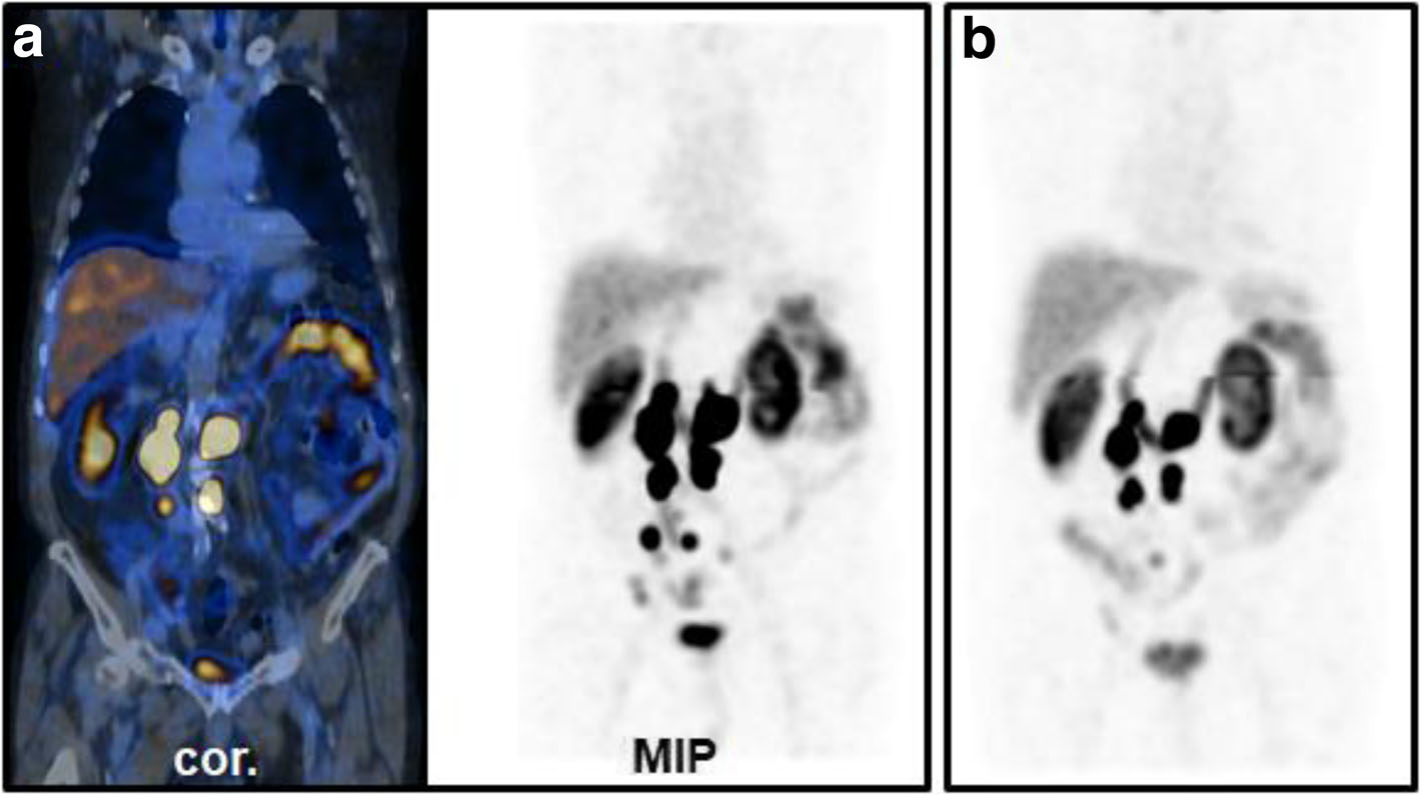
[^99m^Tc]Tc-PSMA-I&S-SPECT/CT of an 82-year-old patient with mCRPC and disease progression under chemotherapy (docetaxel). a Pretherapeutic tumor spread (PSA 137 ng/ml). b Restaging 3 months after the second cycle of ^177^Lu-PSMA therapy (PSA 60 ng/ml)
